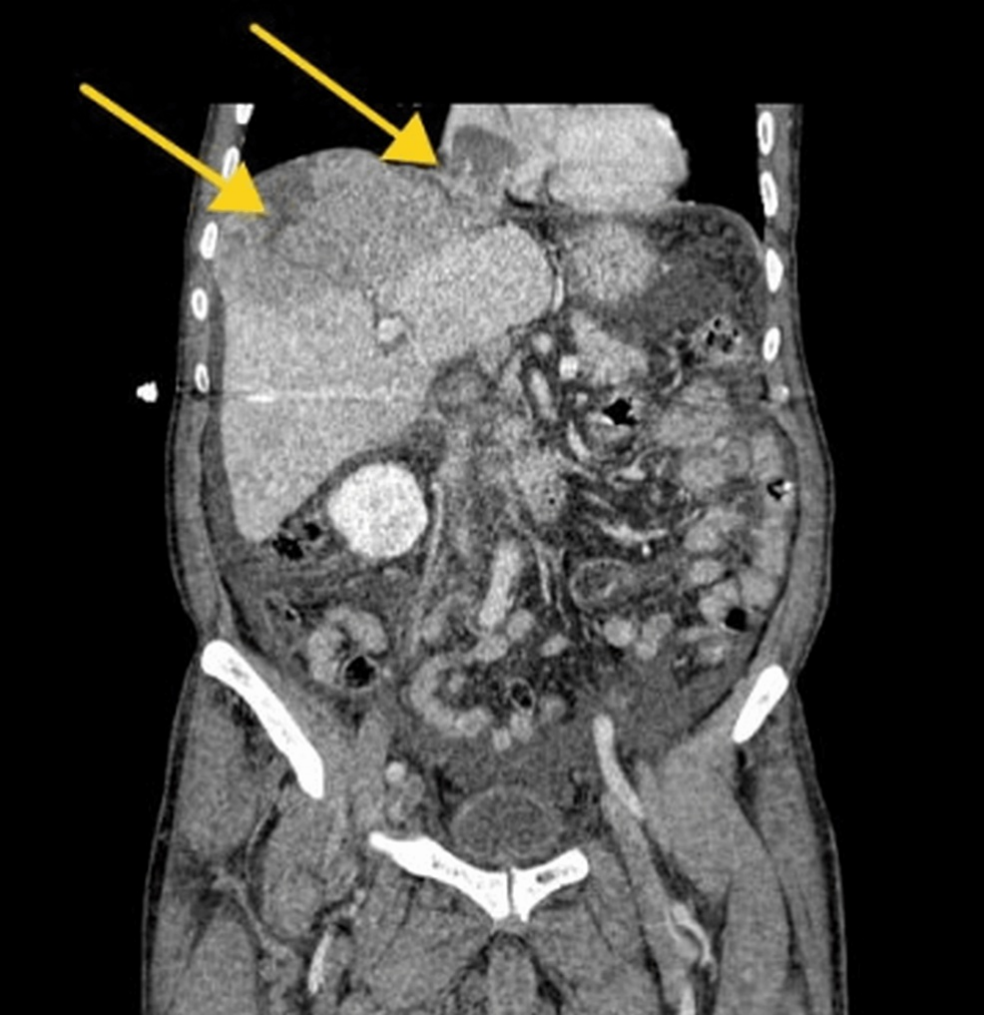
Dynamic hepatic CT scan porto-venous phase coronal reformat image revealing well-defined hypodense hepatic lesion in segments VII and VIII extending to involve the IVC and extending into the right atrium (arrows) CT: computed tomography, IVC: inferior vena cava
